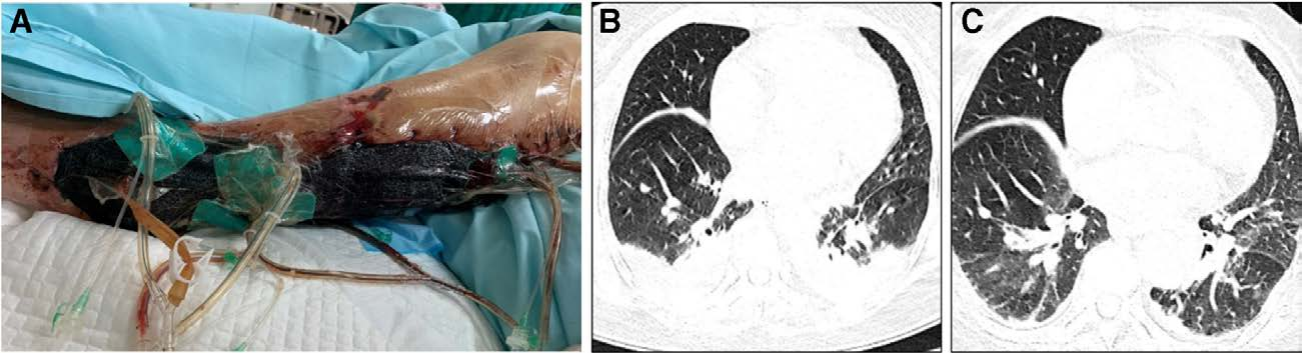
(A) After debridement, we closed the wound with VSD and inserted a drain into the wound. (B) On the second postoperative day, the patient’s chest CT showed patchy shadows in the upper and lower lobes of both lungs and bilateral pleural effusion. (C) On the tenth postoperative day, the CT showed significantly reduced exudate shadows in both lungs and pleural effusion. CT = computed tomography, VSD = vacuum sealing drainage.
